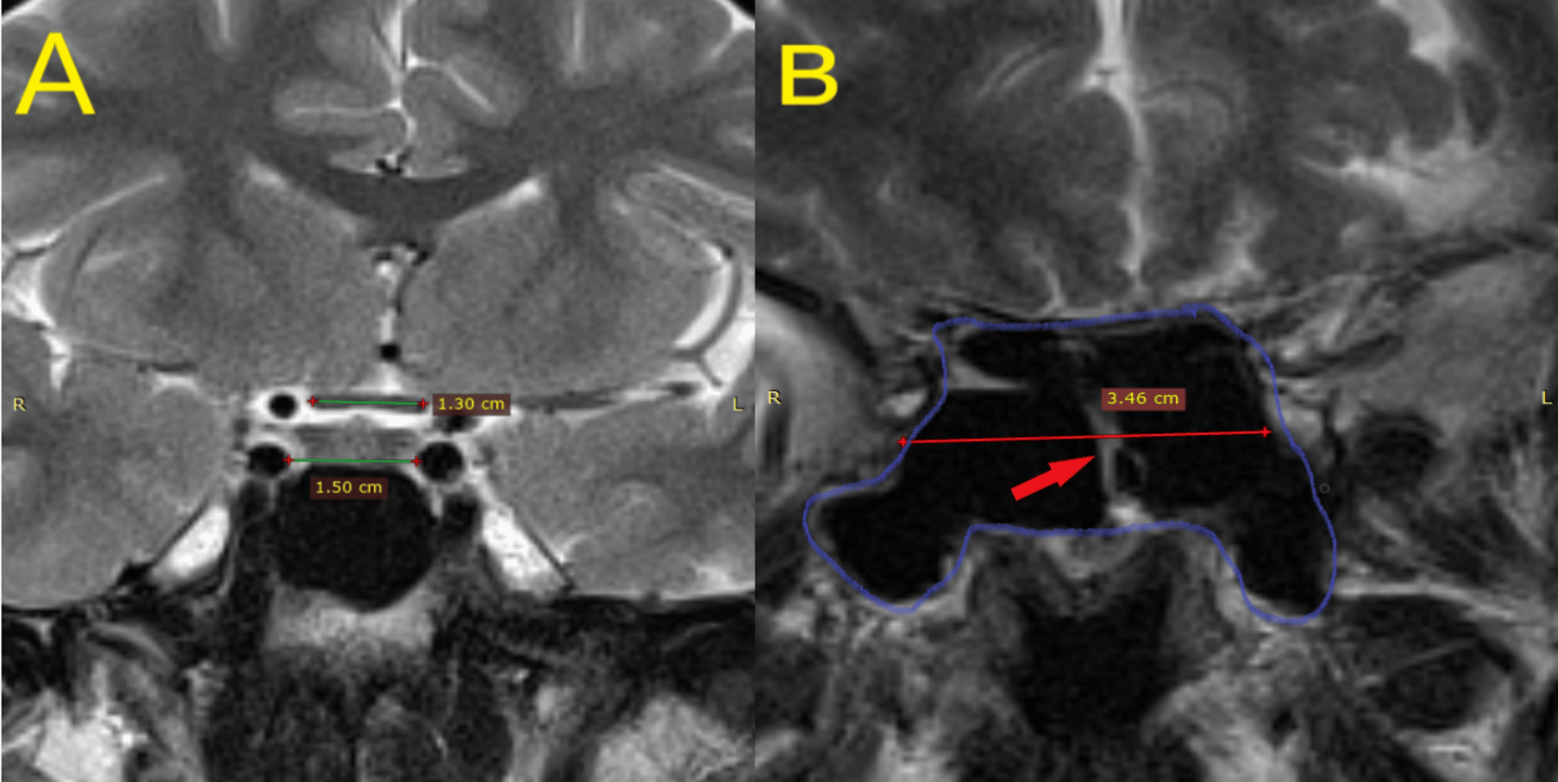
An MRI image of the brain (cortical section in T2 sequence) Panel A demonstrates the measurement of the width of the optic chiasm and intercarotid space. The measurement is taken horizontally across the widest point of each structure; Panel B demonstrates the measurement of the width of the sphenoid sinus (the part circled in blue represents the sphenoid sinus) and the medial septum (red arrow). The measurement of the sphenoid sinus width is taken horizontally across its widest point, while the medial septum's width is measured similarly.
